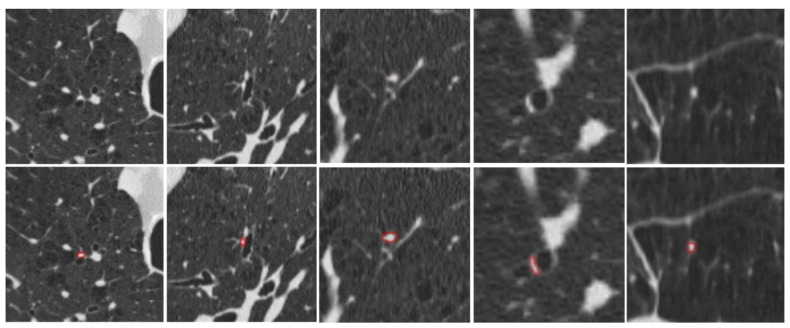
Examples of false positive detections generated by the S-Model. Top row: original CT images. Bottom row: S-Model prediction (red). These errors predominantly stem from small adjacent vessels or airway wall thickening that mimics the density profile of mucus.
